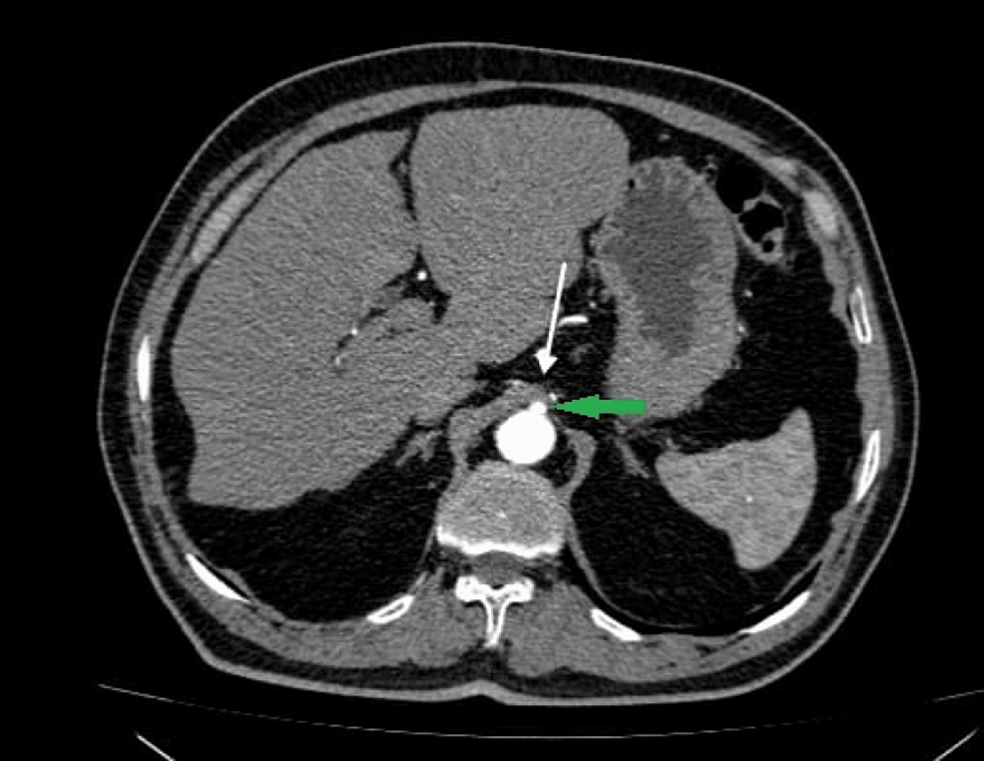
Computed tomography angiography of the abdomen (horizontal view) showing the median arcuate ligament (white arrow) compressing the origin of the celiac trunk (green arrow)
